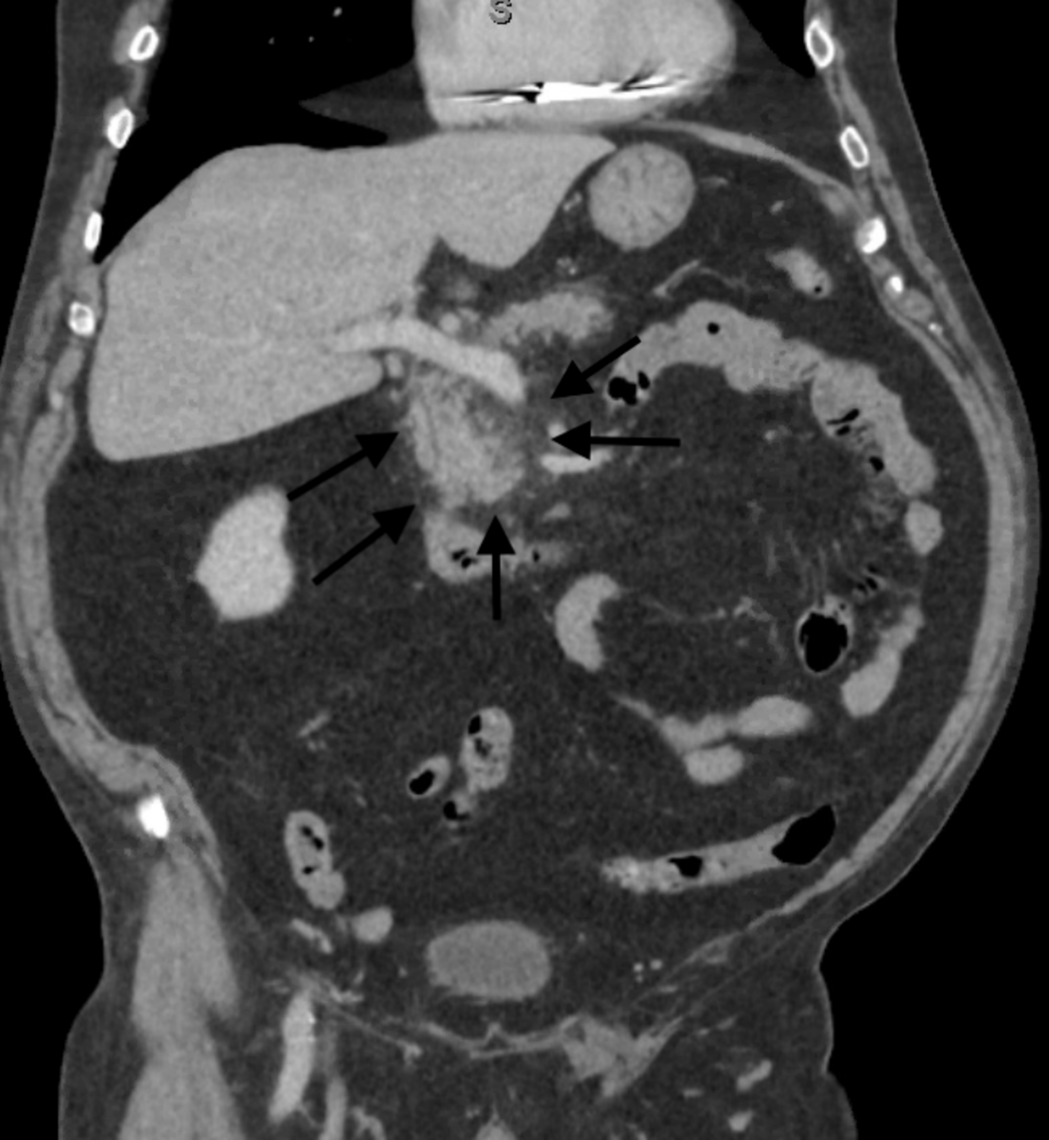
Coronal view of CT scan of abdomen pelvis in porto-venous phase showing fat stranding (black arrows) surrounding the head of the pancreas, compatible with acute pancreatitis CT, computed tomography
